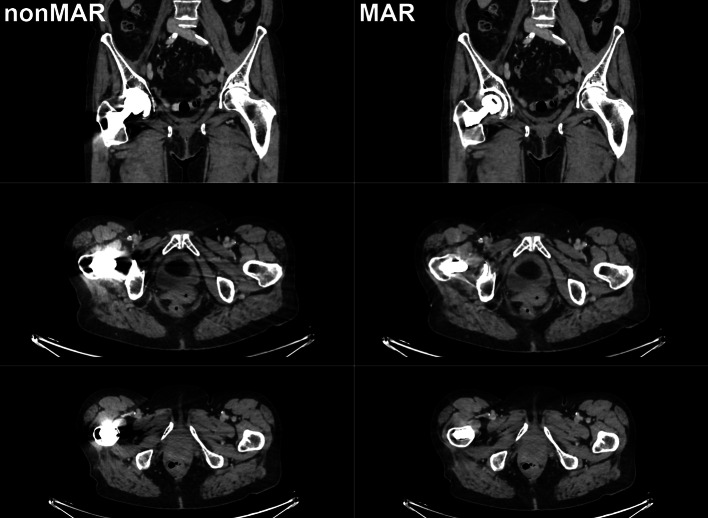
CT images with and without MAR in a patient with unilateral hip prosthesis. Selected coronal (upper row) and axial (second and third row) slices are shown from CT reconstructed without MAR (left column) and CT reconstructed with MAR (right column)
